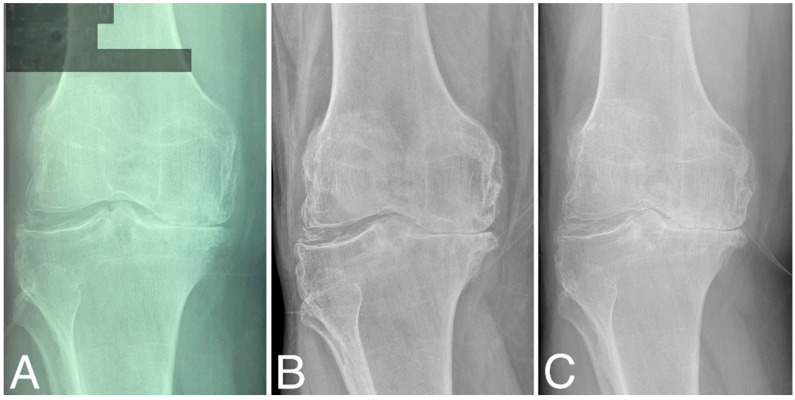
X-ray images of the right knee (A) at baseline, (B) at 60 months time point, and (C) at 84 months time point; all are classified as KL grade 4.
